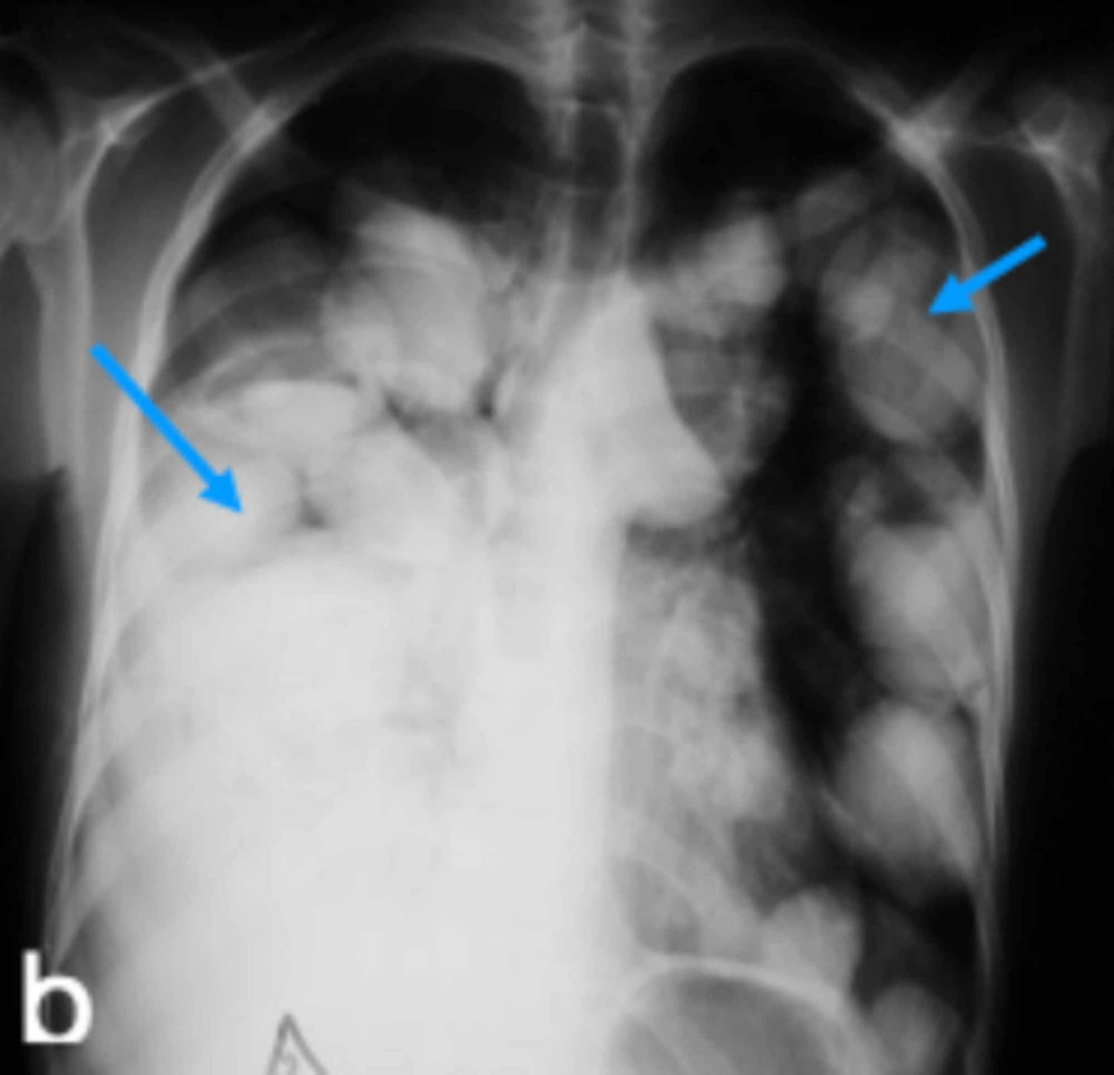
CXR frontal views after drainage of the right pleural effusion revealing multiple bilateral nodules and masses of varying sizes Shorter arrows indicate Echinococcal nodules and the longer arrow indicates decrease in right pleural effusion. CXR: chest X-ray
